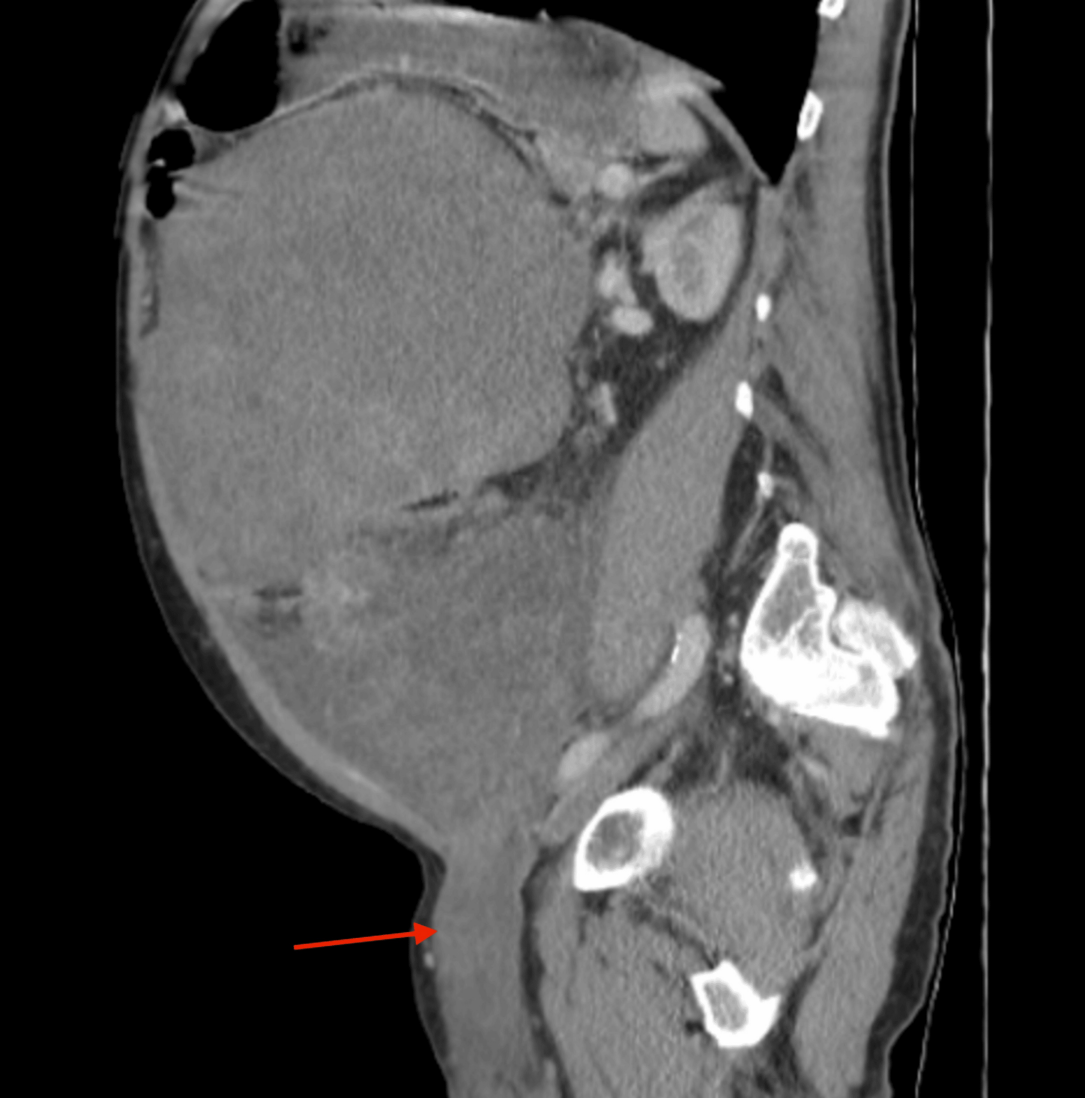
Sagittal CT view of the abdomen and pelvis, showing the large intra-abdominal pelvic mass extending into the left inguinal canal (red arrow) CT: computed tomography
